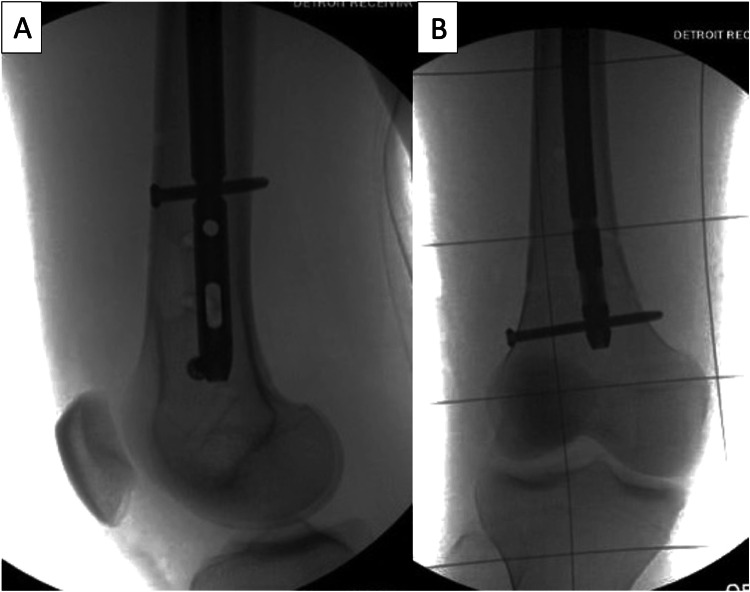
Distal interlocking screws are placed in the previously unused interlocking holes. Once the distal interlocking screw(s) is placed, the rotation is locked in place and all pins can be removed as seen on the lateral (A) and AP (B) radiographs.
